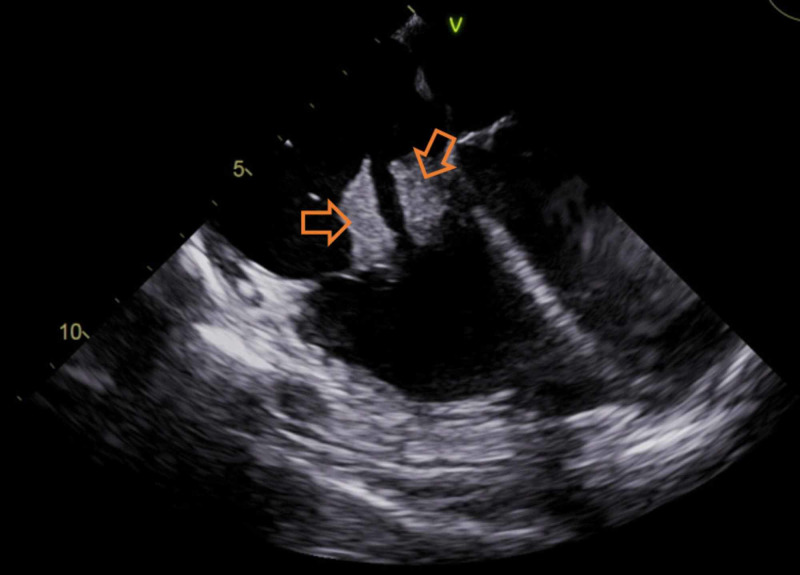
Preprocedural transesophageal echocardiogram (TEE). Multiple large vegatations located on anterior and posterior leaflets of the tricuspid valve prior to percutaneous aspiration procedure.
